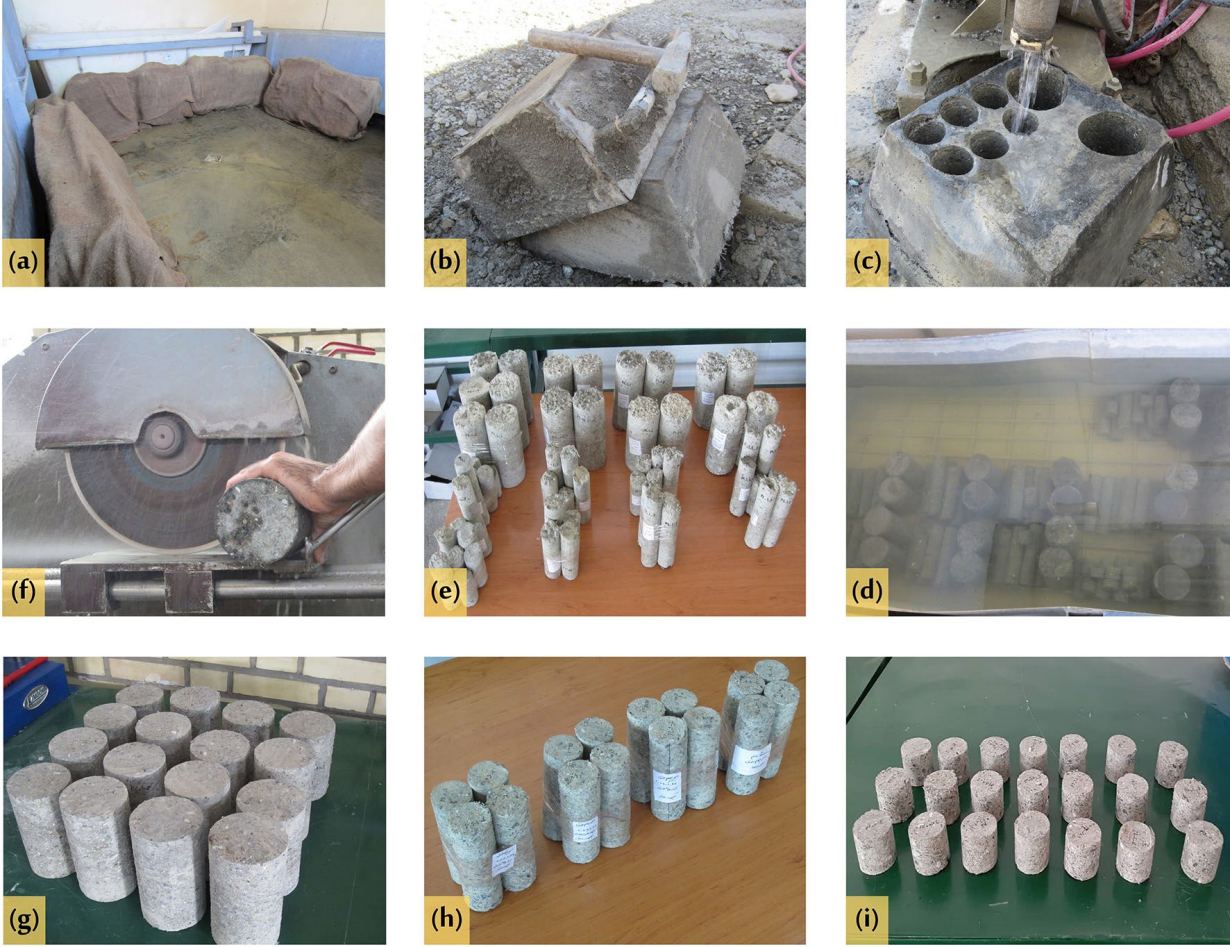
Sample preparation operations, (a) tissue-covered panels in curing room, (b) removing the panels from molds, (c) samples coring operation, (d) immersing the samples in water, (e) transferring the samples to the laboratory, (f) cutting the samples end, (g) prepared samples for uniaxial compressive strength and ultrasonic pulse velocity tests, (h) prepared samples for physical properties tests, (i) prepared samples for splitting tensile strength tests.
